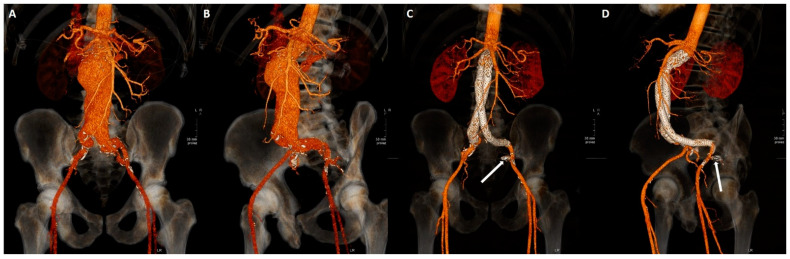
(A–D). Volume rendering reconstructions from CTA before (A,B) and after (C,D) EVAR procedure with left ipogastrigc embolization with coils (arrow).
